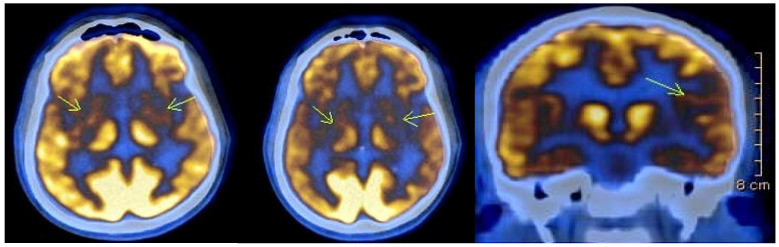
(18)F-FDG PET images of a 35-year-old patient with Huntington’s disease showing severely reduced to absent metabolic activity (indicated with arrows) bilaterally in the striatum. A follow-up scan one year later revealed additional hypometabolism (indicated with arrows) in the left temporoparietal region. Imaging was obtained as part of routine clinical care, and detailed acquisition parameters (e.g., isotope dose and time post-injection) were not available due to the retrospective nature of the data.
